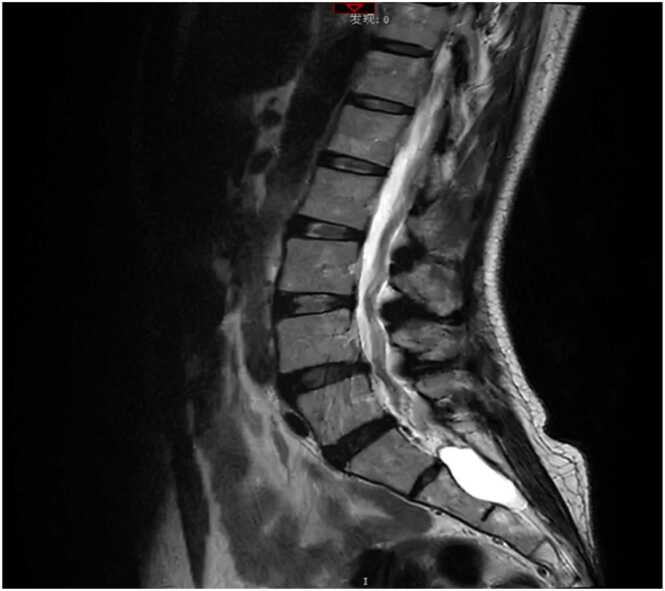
Sagittal lumbar spine T2-weighted MRI: L3/4-L5/S1 disc bulging, lumbar degenerative changes, and a sacral canal cyst.
